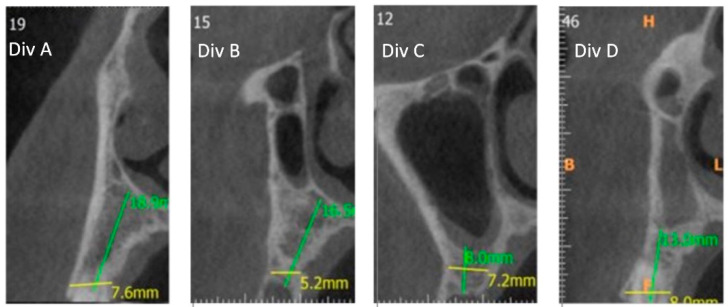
From left to right—CBCT cross sections: Division A (Div. A): Nasal cavity alone; Division B (Div. B): Nasal cavity in adjacent to sinus cavity; Division C (Div. C): Sinus cavity alone above the alveolar ridge; Division D (Div. D): Nasal cavity is positioned superiorly to sinus cavity.
